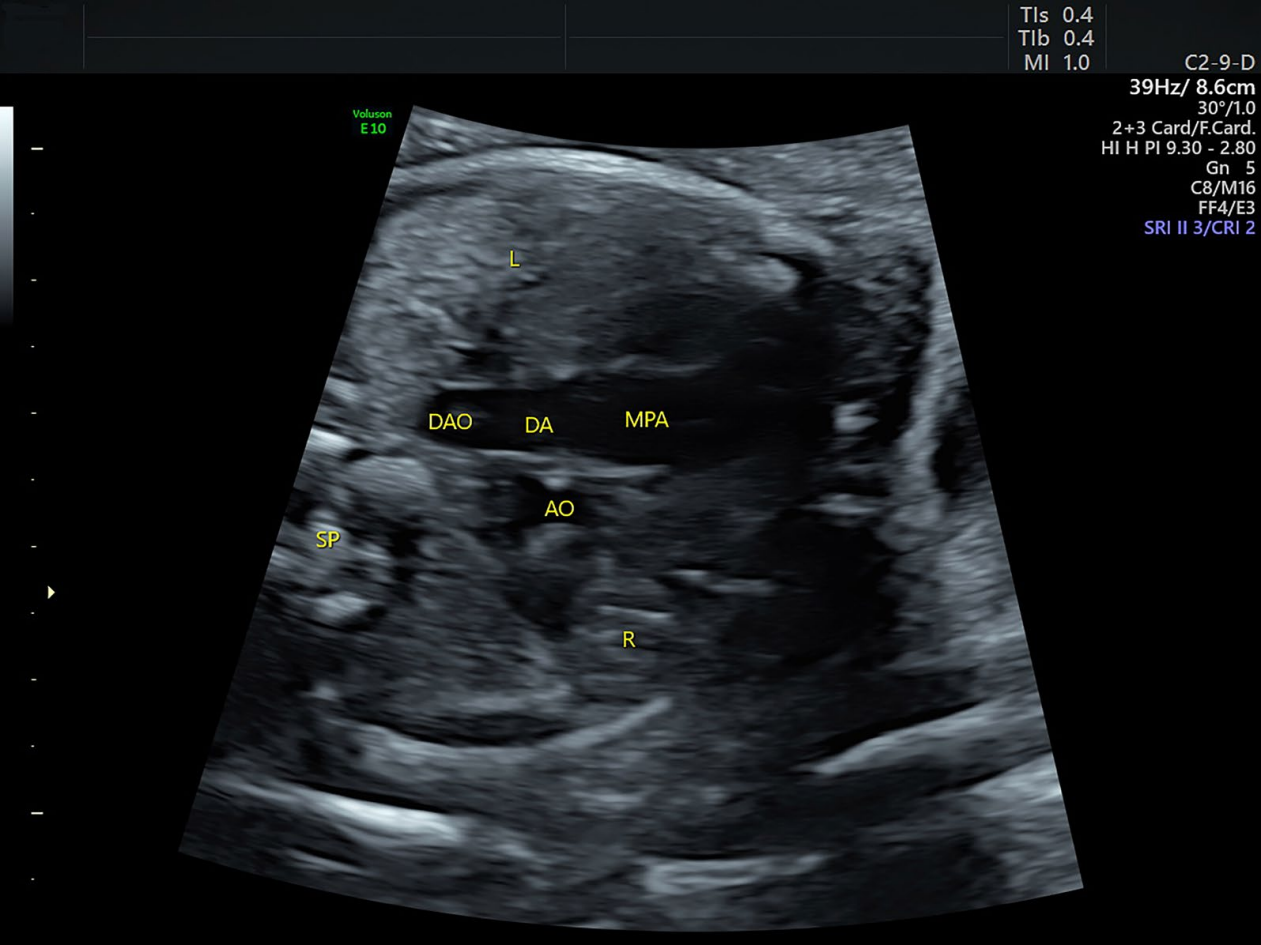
Sonograms of the fetus of coarctation of the aorta at the three-vessel trachea view. The large-sized pulmonary artery is visualized to connect with the descending aorta via the ductus. The aorta is on the right side of the pulmonary artery but is not visualized to drain into the ductus. AO: aorta; DAO: descending aorta; L: left; MPA: main pulmonary artery; R: right; SP: spine
